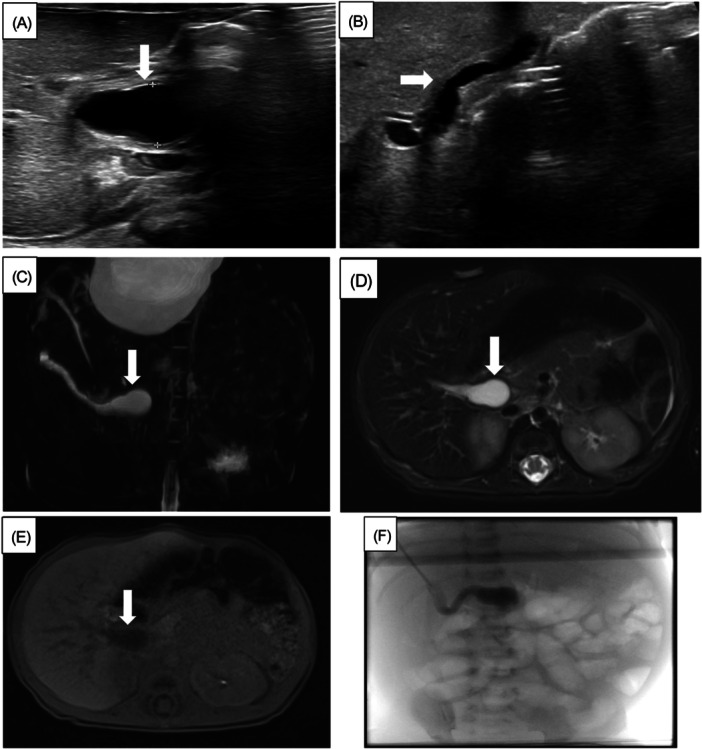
(A, B) Ultrasound Doppler of the liver revealing no dilated intrahepatic biliary ducts with fusiform avascular enlargement of the common bile duct suggestive of Type 1 choledochal cyst (A), and the gallbladder is normal in length measuring 2.5 cm (B). (C) 3D maximum intensity projection image from MRCP demonstrating a fusiform cystic structure in the porta hepatis communicating with the tubular gallbladder. (D, E) Axial T2 fat‐saturated image (D) and axial T1 20 min postcontrast delay image (E) after administration of Eovist. The cystic structure remains dark on T1 and fails to opacify with excreted contrast. (F) Cholangiography revealing patent gallbladder and infrahepatic cyst in the location of the common bile duct with atretic segments at the ampulla as well as the hepatic duct bifurcation, with no bile in the cystic structure, suggestive of cystic variant of BA. 3D, three‐dimensional; BA, biliary atresia; MRCP, magnetic resonance cholangiopancreatography.
